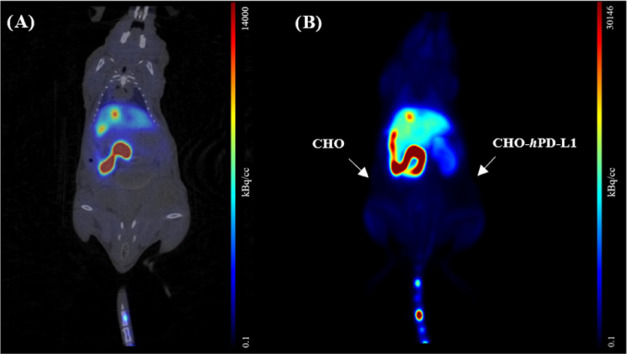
In vivo* distribution of [^11^C]5c during dynamic a μPET/CT
